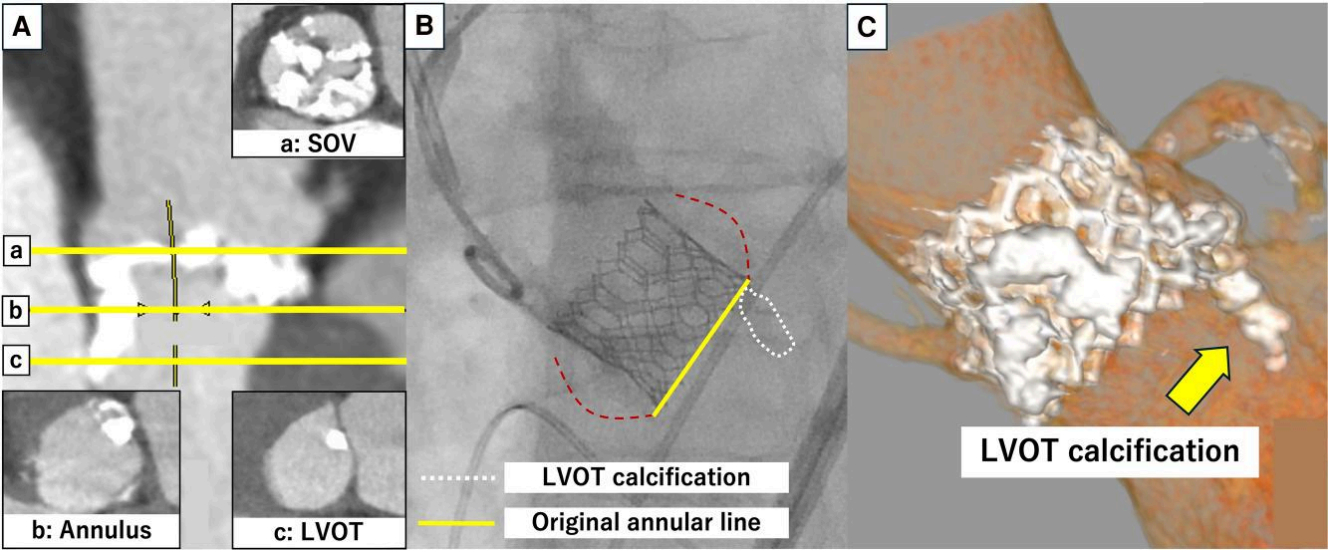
Pre- and post-procedural images of Case 1. (*A*) Pre-procedural computed tomography: a, sinus of Valsalva level; b, annular level; c, left ventricular outflow tract level. (*B*) Aortography after transcatheter aortic valve replacement. (*C*) Post-procedural computed tomography.
